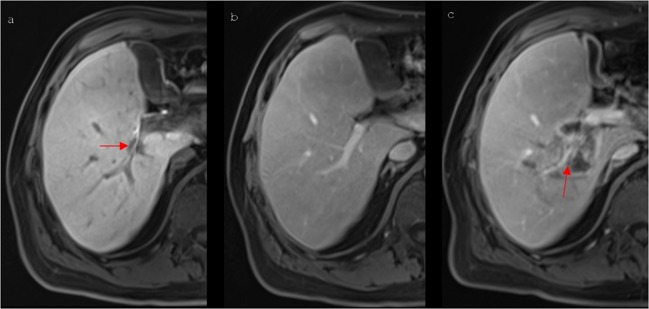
A 64-year old man with a centrally located HCC. (a) Pre-interventional Gd-EOB- DTPA-enhanced T1 vibe 3d fat suppressed magnetic resonance imaging in delayed phase shows a centrally located HCC (arrow) next to the portal vein encasing a bile duct. (b) The corresponding portal venous phase shows a freely perfused right branch of the portal vein. (c) 6 weeks after IRE contrast-enhanced T1 vibe 3d fat suppressed magnetic resonance imaging in portal venous phase shows a newly occurred partial thrombosis (arrow) of the right portal vein.
